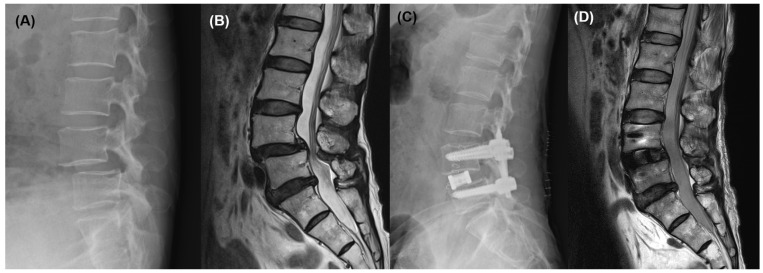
(A) Preoperative lateral radiograph demonstrating grade II degenerative spondylolisthesis at L4–5. (B) Preoperative sagittal MRI showing lateral recess stenosis at L4–5. (C) Postoperative lateral radiograph demonstrating restoration of disc height and reduction in spondylolisthesis after OLIF and posterior percutaneous pedicle screw fixation. (D) Postoperative sagittal MRI obtained 12 days after surgery, showing adequate indirect decompression at L4–5.
